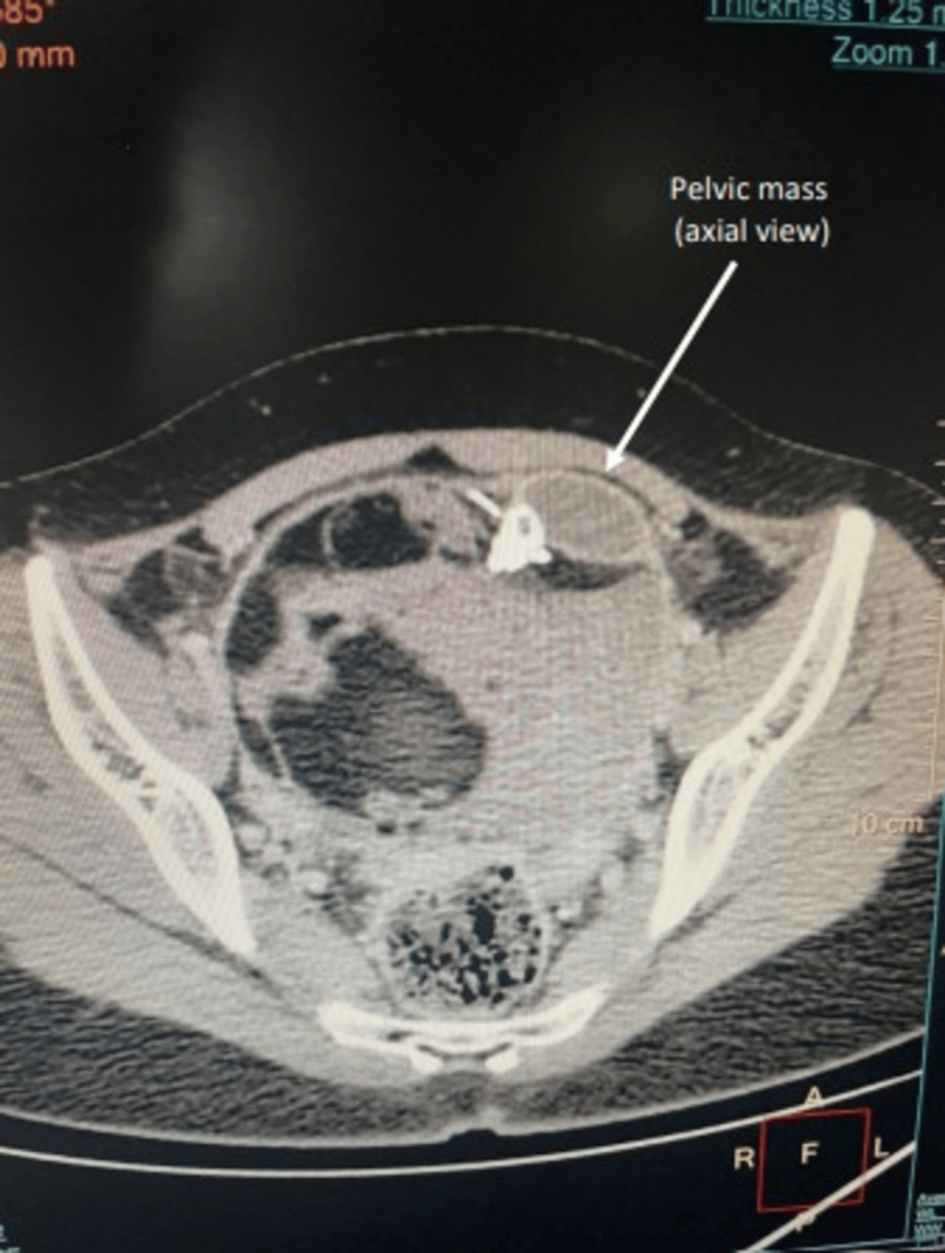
Radiographic contrast-enhanced computed tomography pelvis locating the pelvic mass (axial view) with the 112 x 98 x 118 mm well-defined heterogeneous lesion in focus. White arrow marks the pelvic mass in axial view.
